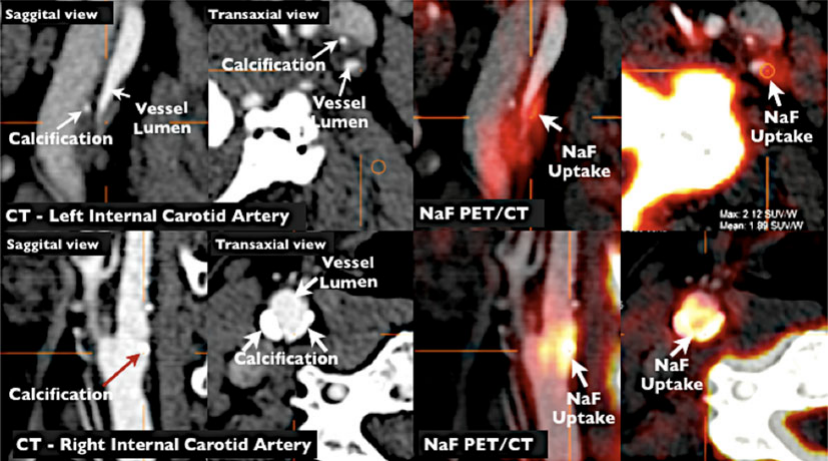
NaF PET/CT imaging of left and right internal carotid arteries of active calcification in a 72-year-old symptomatic patient evaluated at the University of Ottawa Heart Institute. *Upper row* evidence of NaF uptake with a small foci of calcification on CT in the left internal carotid symptomatic culprit vessel. There is a mismatch between the region of NaF uptake and calcification on CT. *Lower row* Evidence of calcium nodules with matched NaF uptake at the right internal carotid artery
